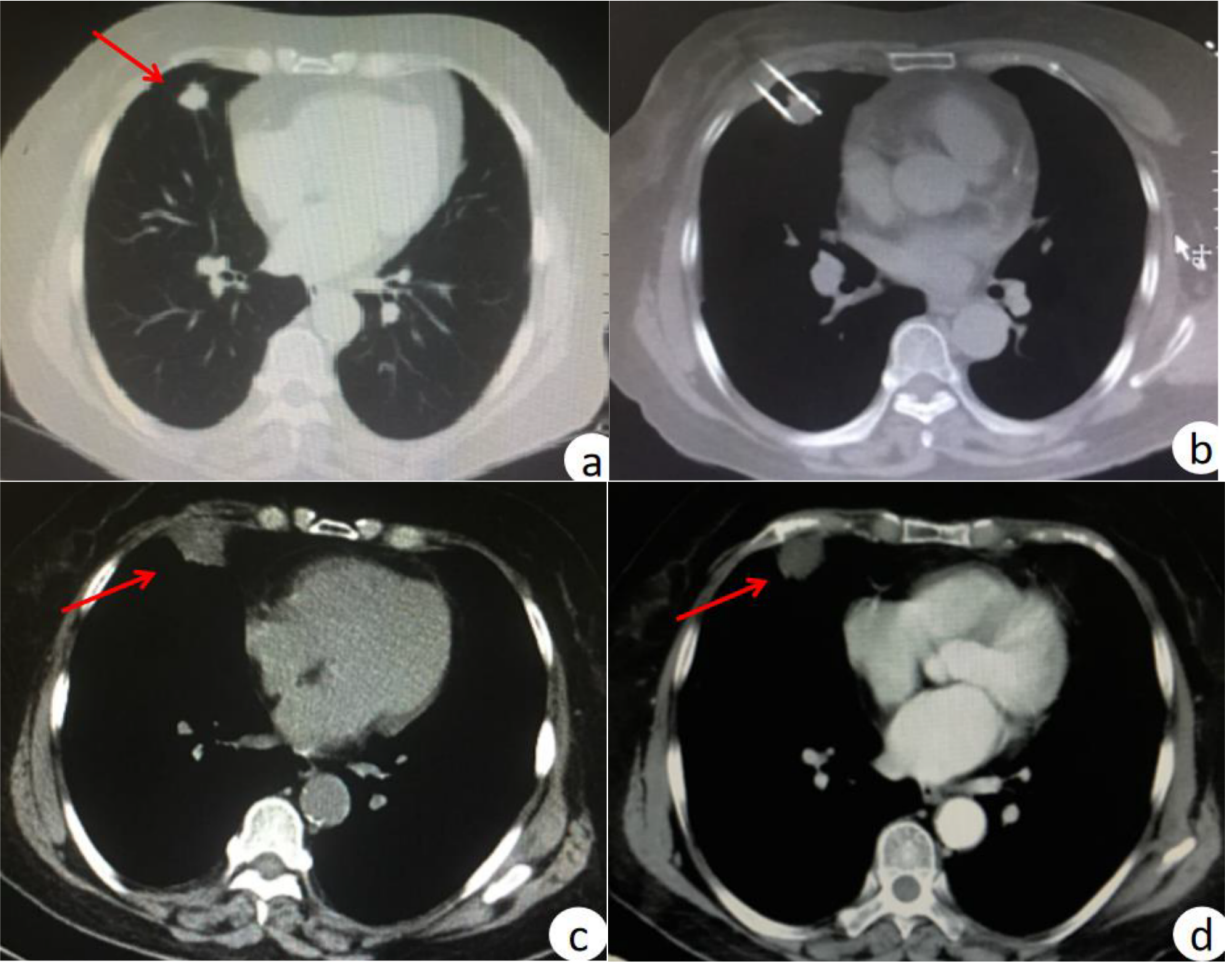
CT-guided MWA treatment for stage I non-small cell lung cancer. (a) before MWA treatment; (b) CT scan during MWA; (c) after MWA, the halo shadow was significantly larger than the lesion; (d) enhanced CT scan at 3 months post-MWA treatment showed no active lesions.
